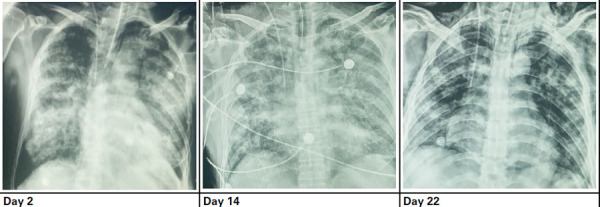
Chest radiograph images during the patient's hospitalisation.
